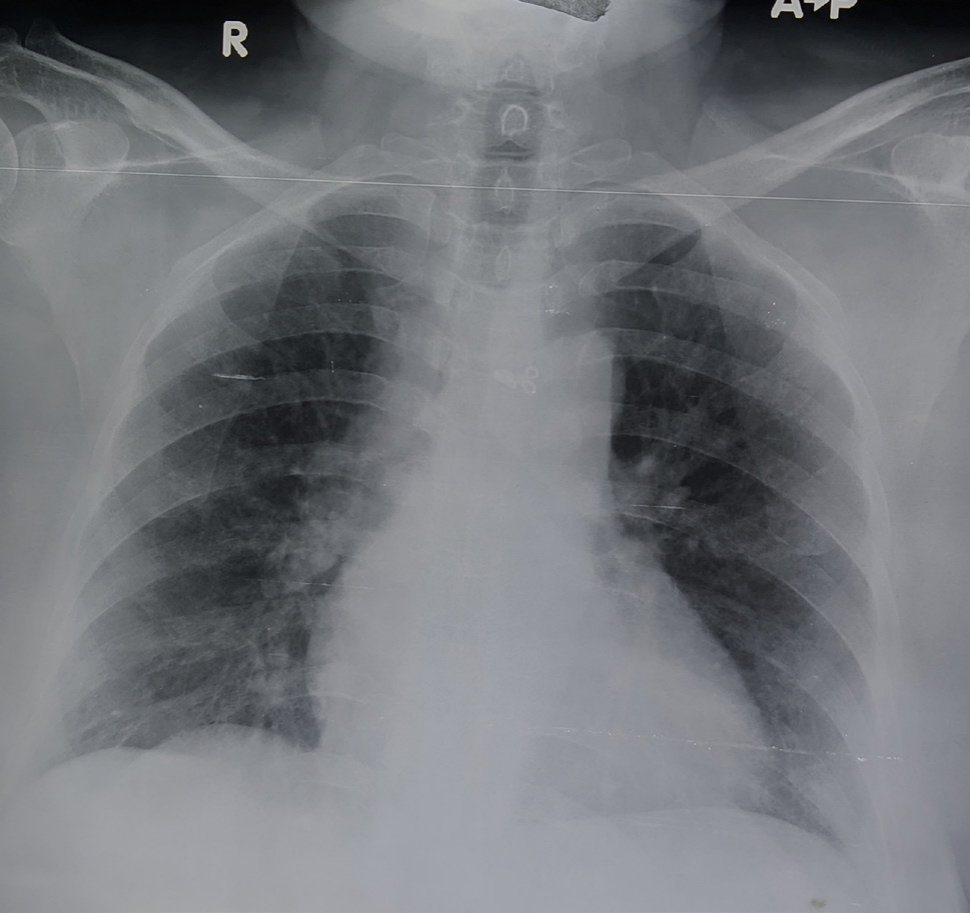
Chest X-ray (AP View) during discharge.
